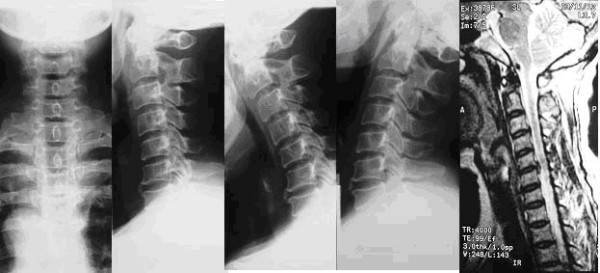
53 y, female, CSM. Preoperative anteroposterior and lateral x-rays show straight alinement and degeneration. Lateral flexion/extension x-rays show the range of motion before operation. And sagittal MRI T2 show that extensive disc degeneration in cervical disc. Hight loss of disc space and spinal cord compression can be found in C5/6.
